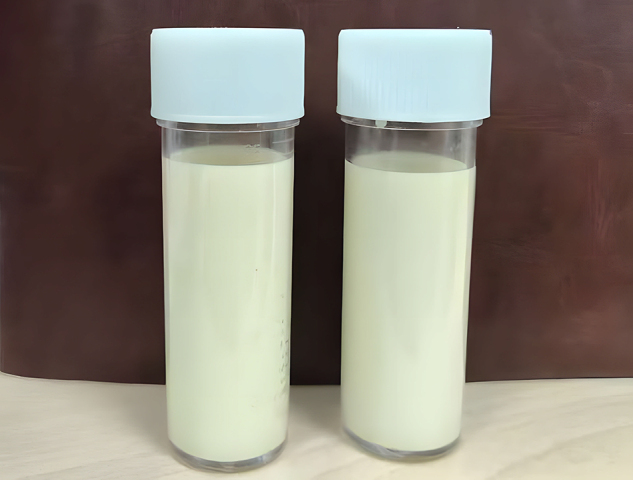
Pre-operative CT scans of mesenteric cysts. Pre-operative CT scan demonstrating multiple cystic lesions in the right abdomen and pelvis, with the largest lesion measuring 22.34 cm × 13.89 cm, characteristic of mesenteric cysts in the present case.
